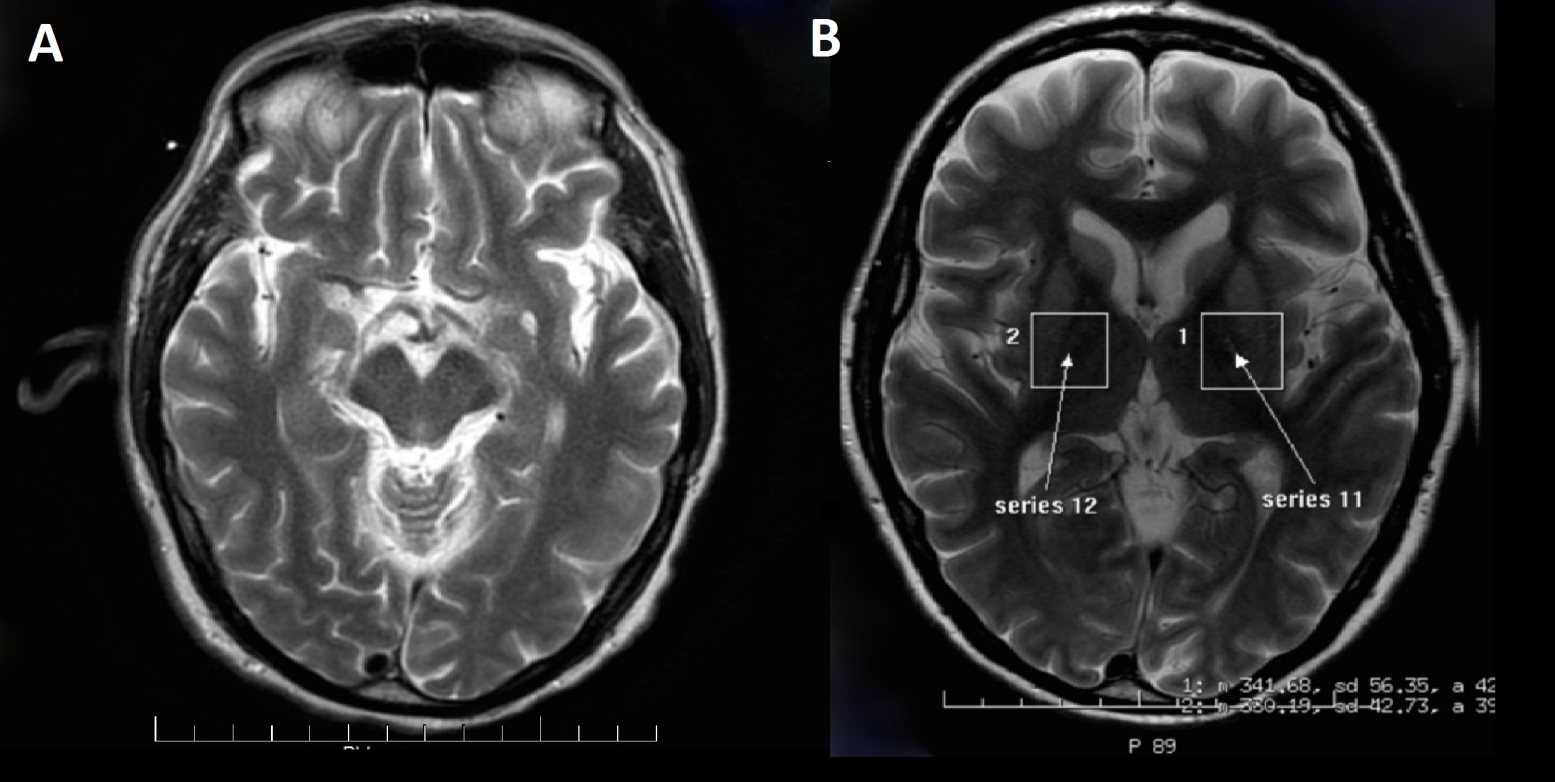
Diffusion weighted imaging (DWI) MRI [A, B] Shows extensive signal abnormality in the bilateral basal ganglia and cerebral cortex
